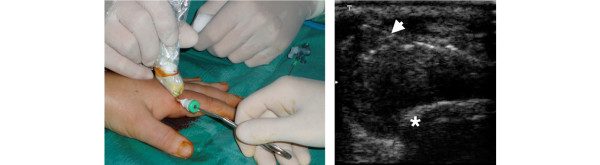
Ultrasound-guided synovial biopsy of a second metacarpophalangeal joint using the portal and forceps technique. Arrow, open forceps inside the joint; asterisk, metacarpal hand.
